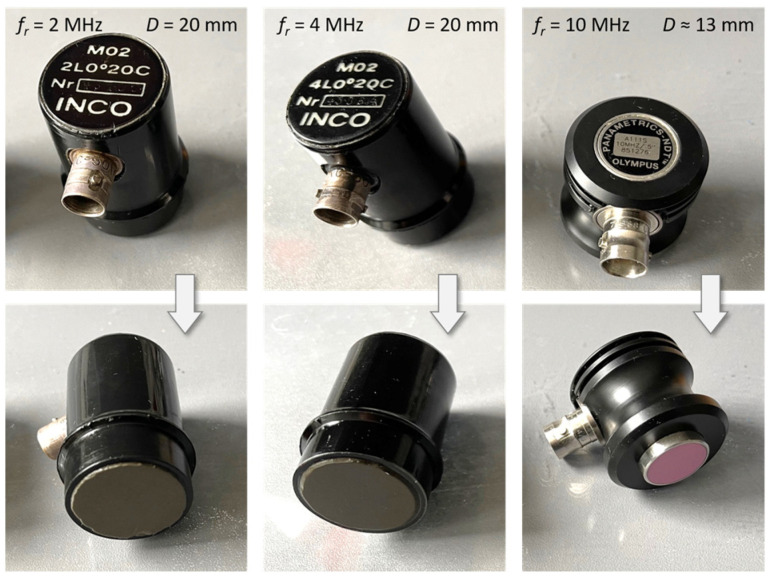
Ultrasonic sensors used for measurements.
